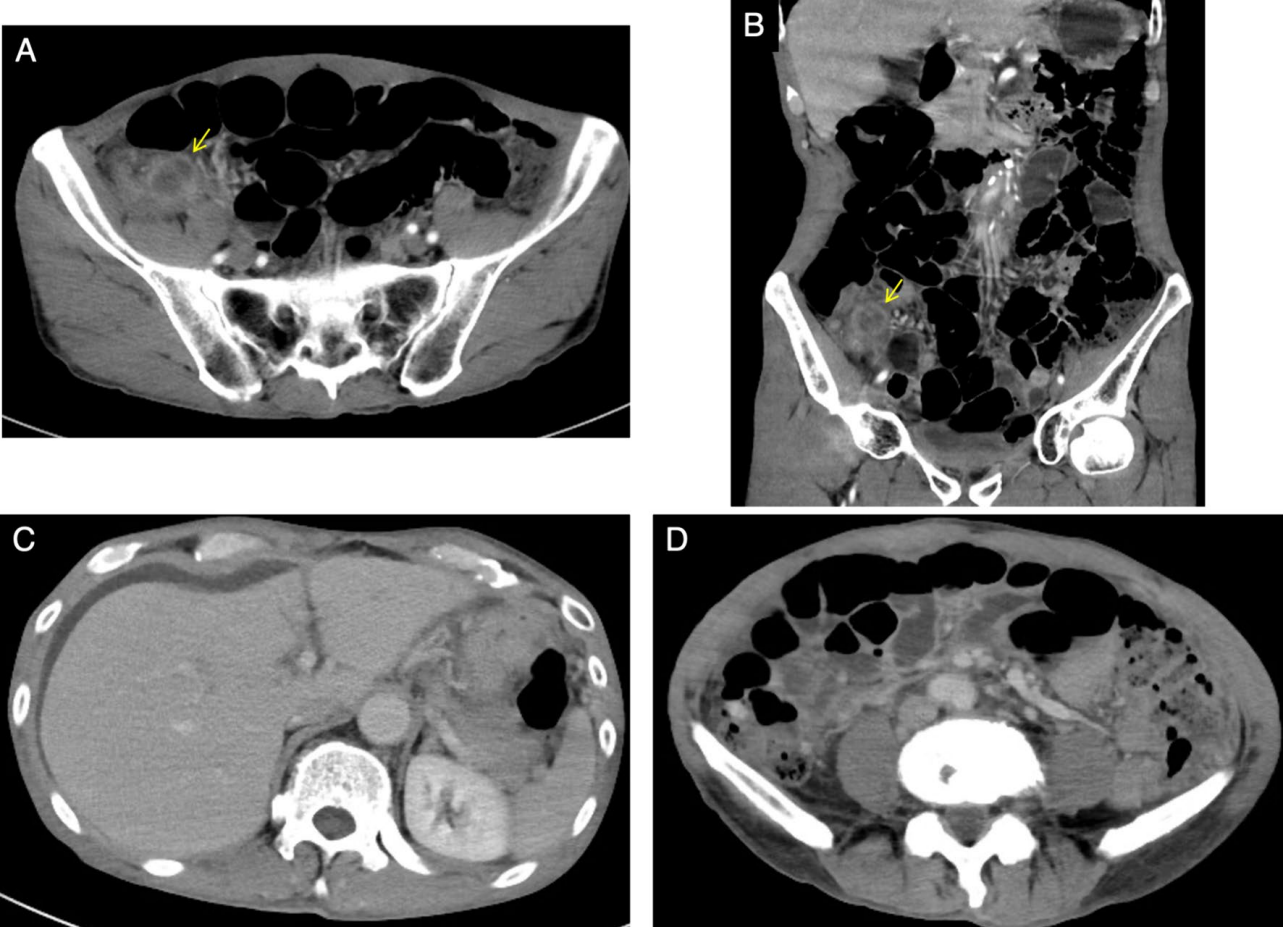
Abdominal enhanced CT findings. A, B CT showing appendicular abscess. Yellow arrows show appendicular abscess. C CT showing peritoneal thickening and ascites. D CT showing an ileal abscess. *CT* computed tomography
